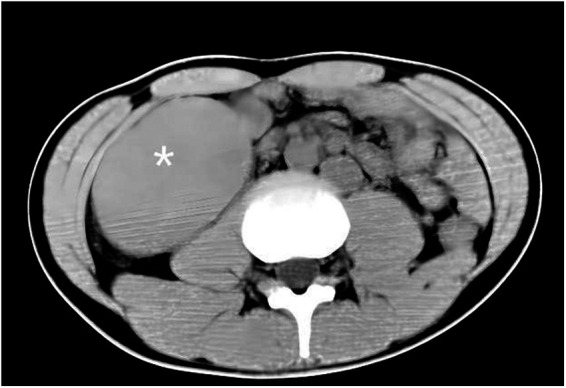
Coronal section CT scan of abdomen. Organ inversion, splenomegaly, and intestinal effusion-labeled spleen.
